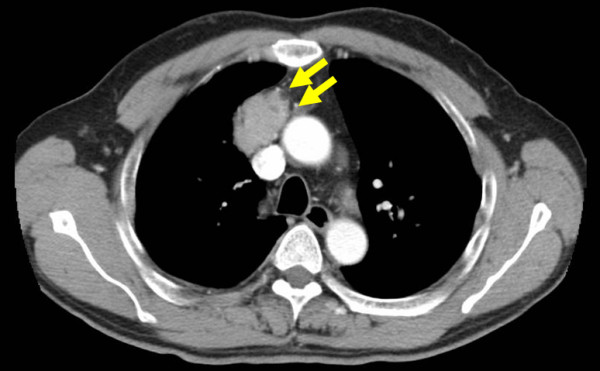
Enhanced chest CT scan revealing a 42-mm-sized solid mass with an unclear margin (arrows) with the normal thymus in the anterior mediastinum.
